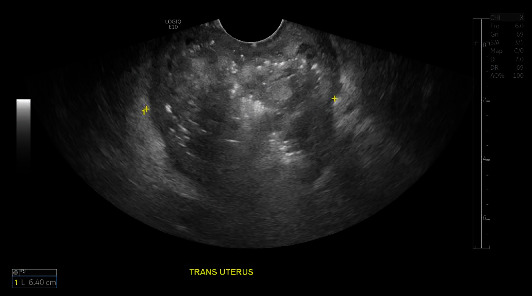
Transvaginal ultrasound performed in the emergency department. Foul-smelling discharge noted in addition to heterogeneous material and air within the uterus.
